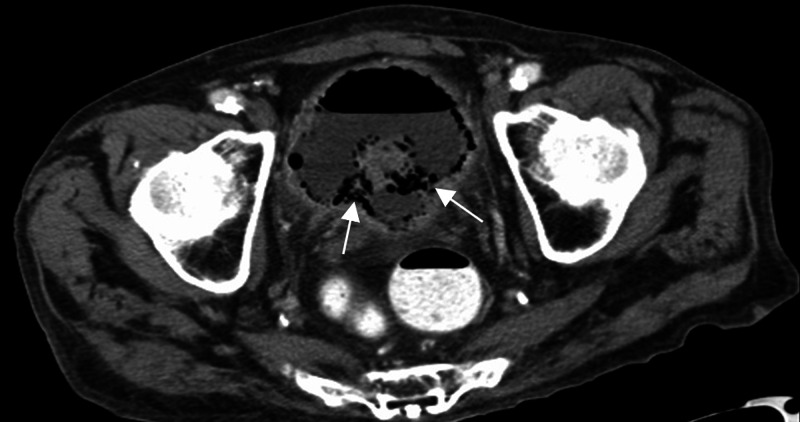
CT abdomen and pelvis axial section through the urinary bladder Diffuse bladder wall thickening with air seen circumferentially within the bladder wall (arrows).
